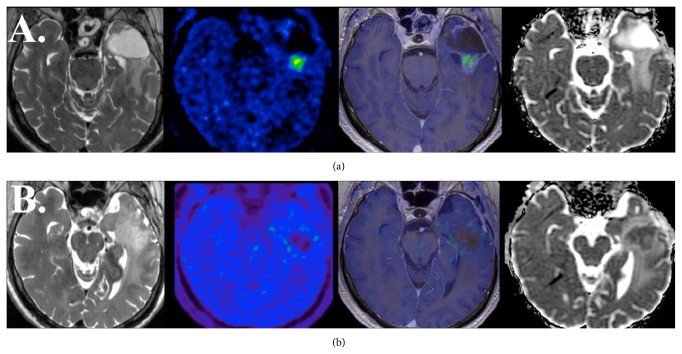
Single modality FMISO PET/MR imaging in a 65-year-old man with recurrent left temporal lobe WHO grade III anaplastic astrocytoma immediately prior to (a) and concurrent with bevacizumab therapy (b). Baseline, simultaneously obtained, axial T2 weighted (left), FMISO PET (middle left), fused FMISO PET and T1 weighted post contrast (middle right), and apparent diffusion coefficient (right) PET/MR imaging demonstrate recurrence of disease evidenced by contrast enhancing focus bordering the posterior margin of an anterior left temporal lobe resection cavity. This region demonstrates increased FMISO uptake with no evidence of associated reduced diffusion. Follow-up PET/MR imaging 10 weeks later (after 4 doses of bevacizumab) demonstrates the development of a nonenhancing T2 hyperintense mass about the posterior margin of the resection cavity that is associated with the development of reduced diffusion and diminished FMISO uptake.
